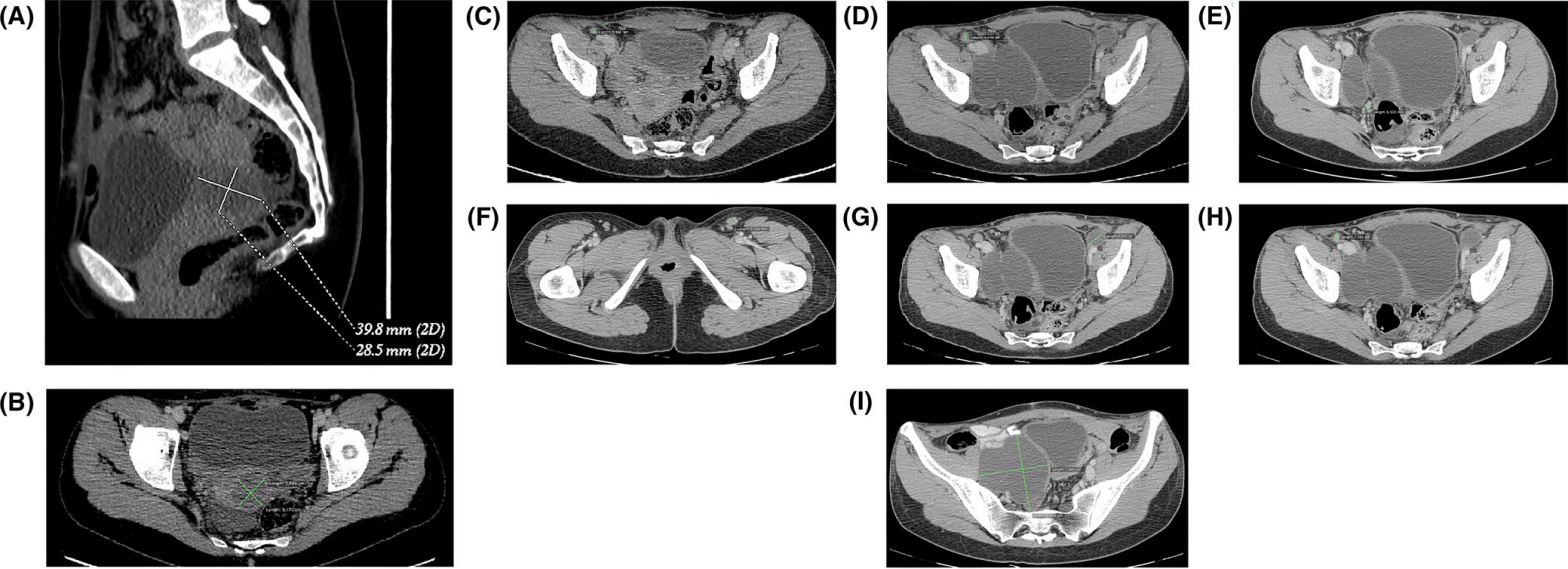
Contrast‐enhanced computed tomography (CT) scans performed on 15 October 2014 and 24 March 2015. (A and B) CT scan performed on 15 October 2014 after the first surgery shows a residual tumor. (C and D) Pelvic CT scan shows lymph node size growth from 0.6 cm (15 October 2014) to 0.9 cm (24 March 2015). (E, F, G, and H) Enlarged lymph nodes with the largest diameter of 2.2 cm (24 March 2015). (I) Bilateral lymphoceles (24 March 2015)
